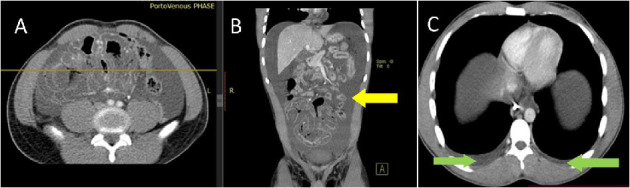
Contrasted abdominal pelvic CT scan. (A) Axial and (B) coronal reformatted showing extensive nonenhancing oedematous mural wall thickening involving the ileal loops with foci of pneumatosis intestinalis and an ulcerative component at mid-distal ileum. Note also gross ascites (yellow), no evidence of mesenteric vessel thrombosis. Mild bilateral pleural effusions (green) more in the right as seen in (C).
